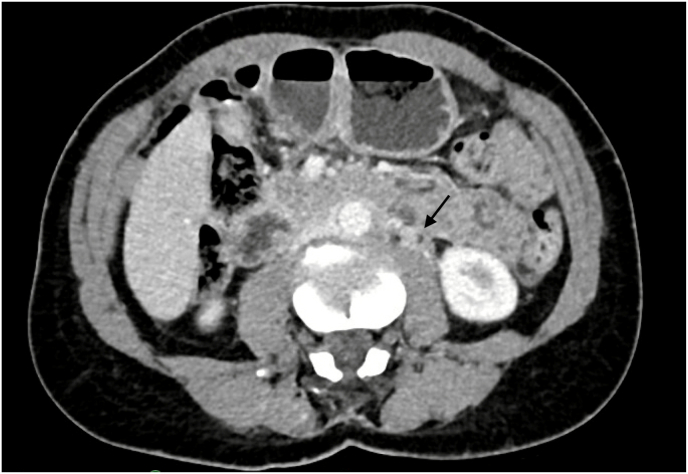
CT Urogram with arrow pointing to urothelial thickening in proximal ureter.
